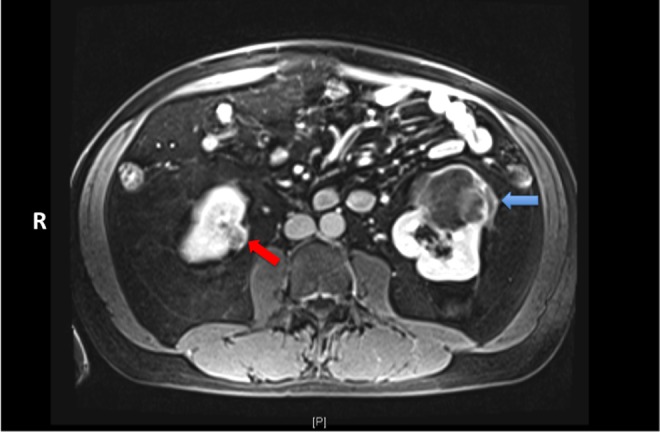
Axial cut of a contrast-enhanced MRI showing a 7.0 × 7.3 × 5.2 cm anterior, mid-to-lower pole left renal mass (*blue arrow*) and a 1.5 × 1.9 × 1.6 cm medial lower pole right renal mass (*red arrow*).
